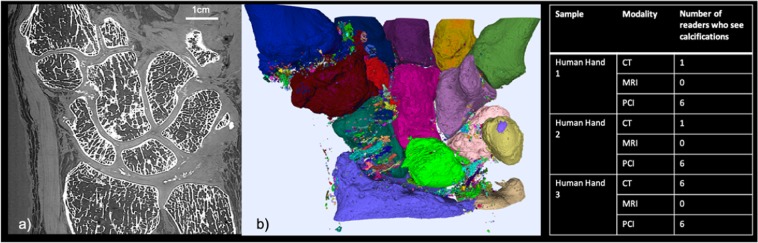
PCI-CT for chondrocalcinosis detection: (a) PCI-CT coronal slice from the severe chondrocalcinosis hand case. (b) 3D volume rendering of the segmented calcified structures from the PCI volume. The juxtaposed table summarized the number of readers that detected calcifications from each sample dataset.
